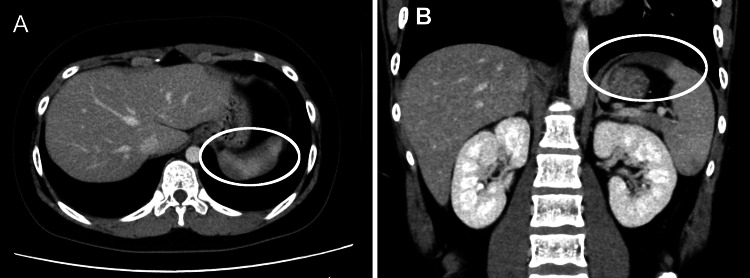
Axial (A) and coronal (B) contrast-enhanced CT images showing splenic infarction A well-demarcated, wedge-shaped hypoenhancing area is seen in the spleen (white circles), consistent with splenic infarction.
